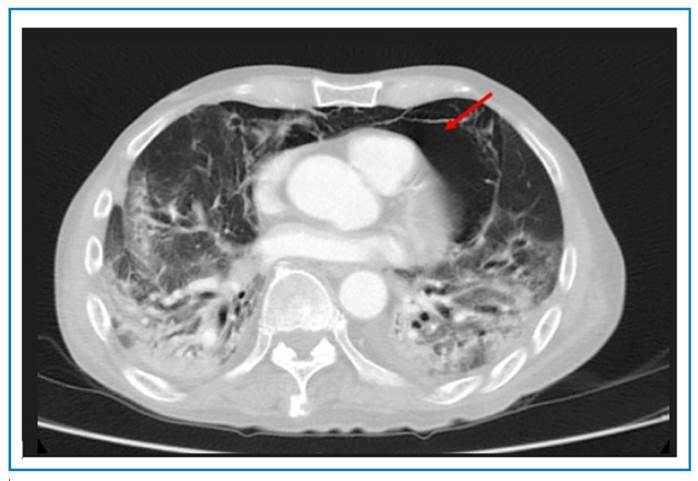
Computed tomography (CT) of the thorax with pneumomediastinum and pneumopericardium shown by the red arrow.
